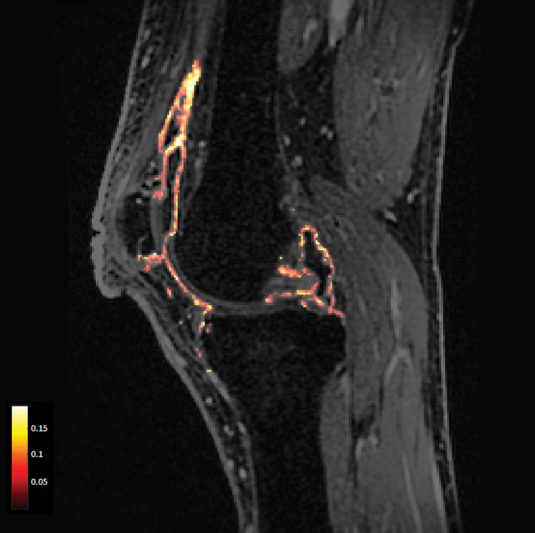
Sagittal magnetic resonance dynamic contrast-enhanced magnetic resonance image with a map of K
